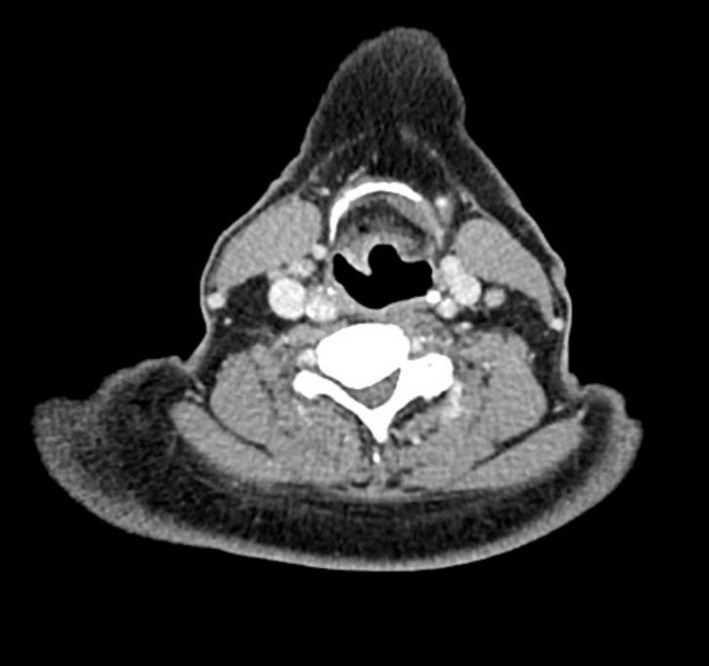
Axial slice of CT of head and neck showing subtle right‐sided thickening of the caudal aspect of the epiglottis
